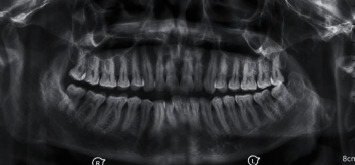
Postoperative panoramic radiography showing excision of the lesion and free of recurrence 1 year after surgery.
